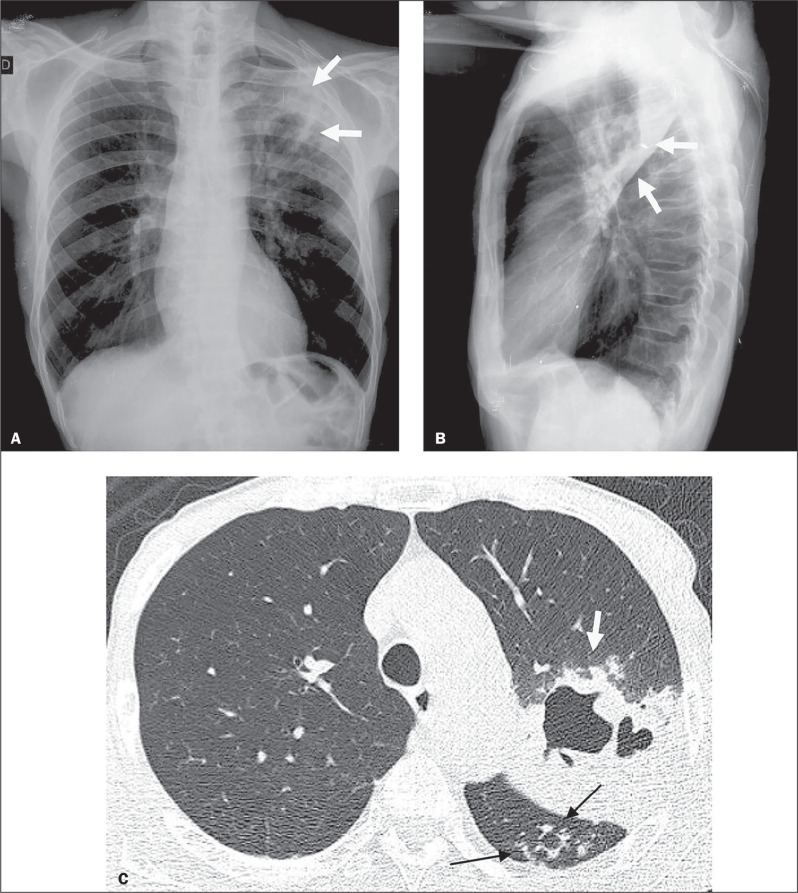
Chest X-ray (A,B) and HRCT (C) of a 66-year-old male with pulmonary
